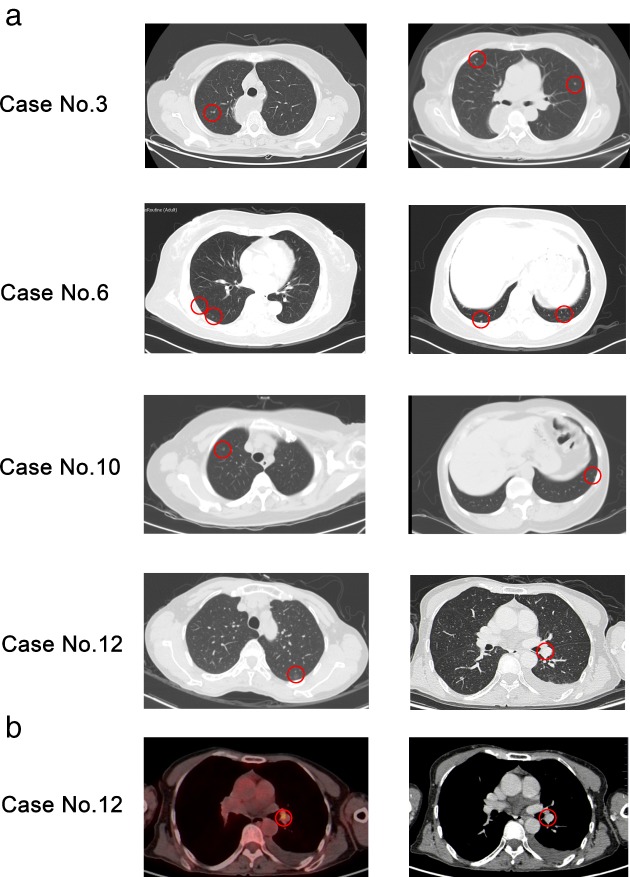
(a) Thin‐section chest computed tomography scan (1 mm collimation) demonstrated multiple well‐defined nodules of various sizes in four patients. (b) Preoperative 18‐fluoro‐2‐deoxy‐D‐glucose positron emission tomography examination revealed an increased maximum standardized uptake value of 4.8 in one patient. Red circles indicate pathological representation of minute pulmonary meningothelial‐like nodules.
